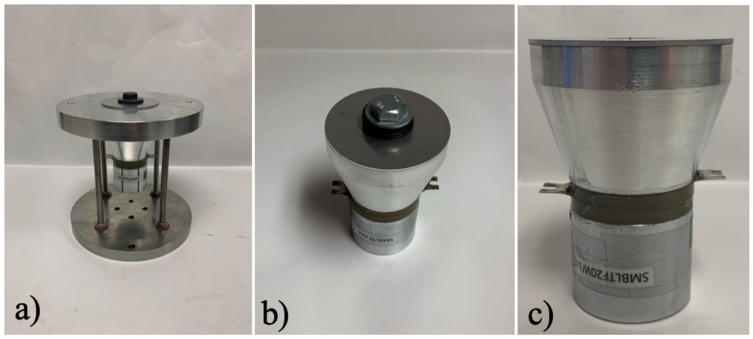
Images of ultrasonic implementation setups. (a) Transducer connected to the aluminum build plate suspended by four rods with a thin plate connected to its surface via a bolt. (b) A thin plate securely bolted to transducer surface. (c) A thin plate superglued to the transducer surface.
